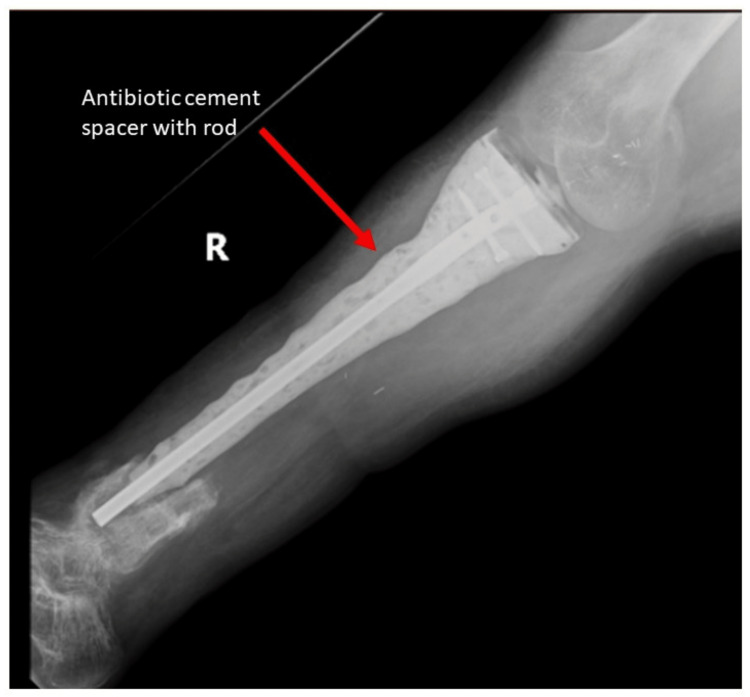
Radiograph after resection of tumor and placement of spacer.
